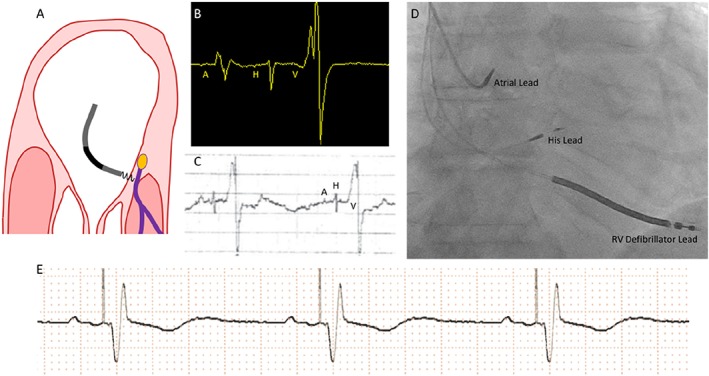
(A) Intended area within right atrium for implantation of the His pacing lead. The target area is where the non‐branching part of the His bundle sits above the tricuspid valve on the right atrial—left ventricular part of the membranous septum. (B) Appearance of a single cardiac cycle recorded on an EP system, from a well‐positioned His lead. Note that the atrial component (A) is less than half the size of the ventricular component (V). There is a His signal (H) between the two. This appearance suggests a favourable location. (C) The electrogram can also be displayed using the Pace–Sense Analyser. It is advisable to measure initially a unipolar signal utilizing the atrial channel with a gain setting of 0.05 mV/mm and a sweep speed of 50–100 mm/s. The ventricular channel is not sufficiently sensitive to detect His potentials. (D) This anterior–posterior projection radiographic image shows the typical anatomical location for the His lead (labelled). (E) Rhythm strip showing successful His‐bundle pacing. RV, right ventricular.
